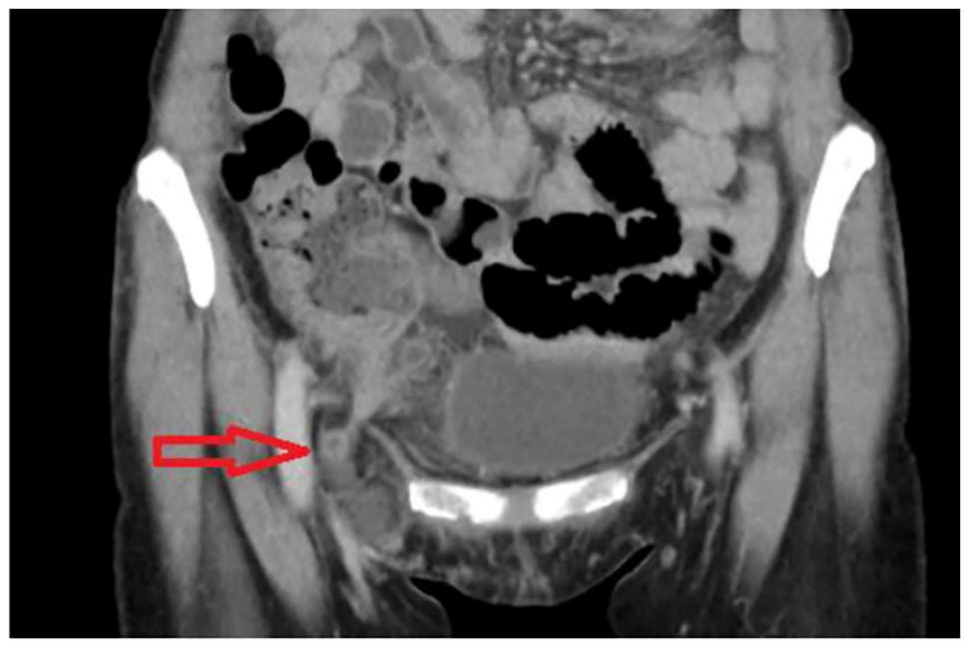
Coronal plane of multidetector computed tomography image. Another plane of entrapped appendix and fat stranding at right lower abdomen
